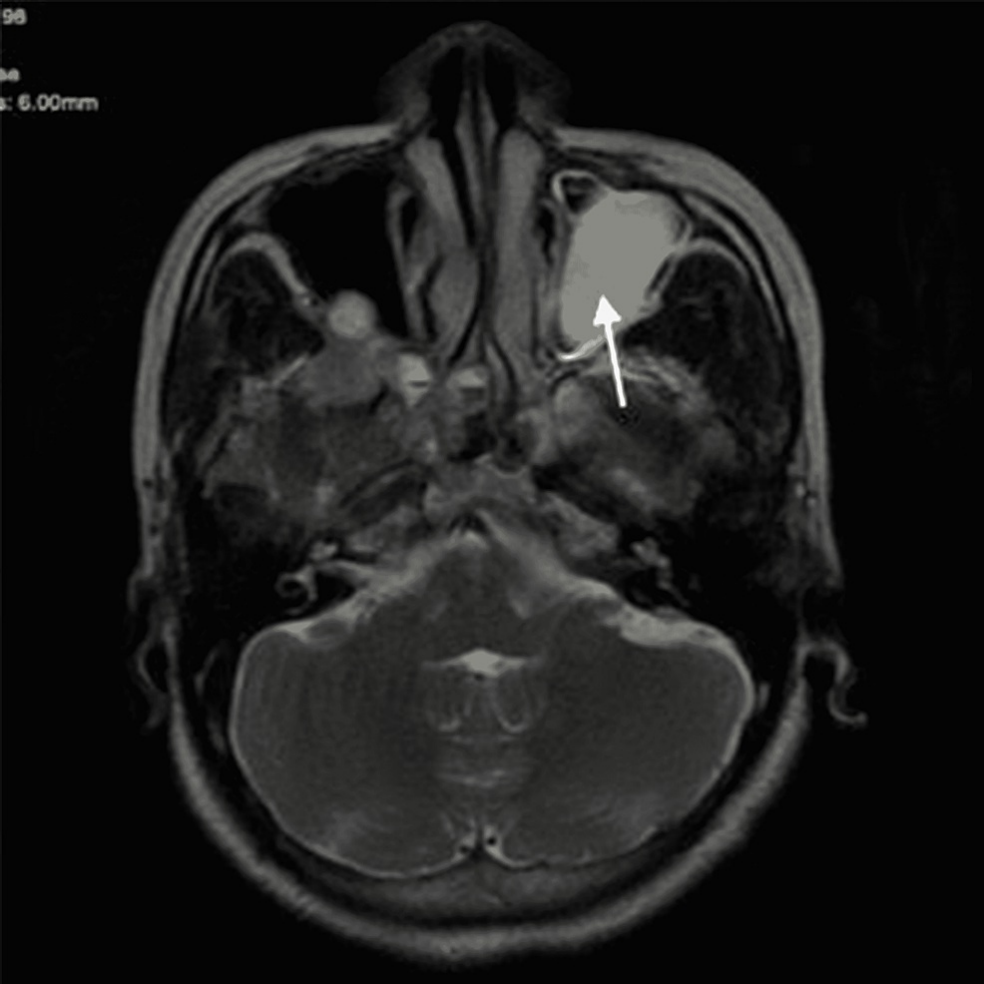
MRI scan of brain and orbit Arrow indicating enhancing lesion in the retrobulbar aspect of orbital apex
